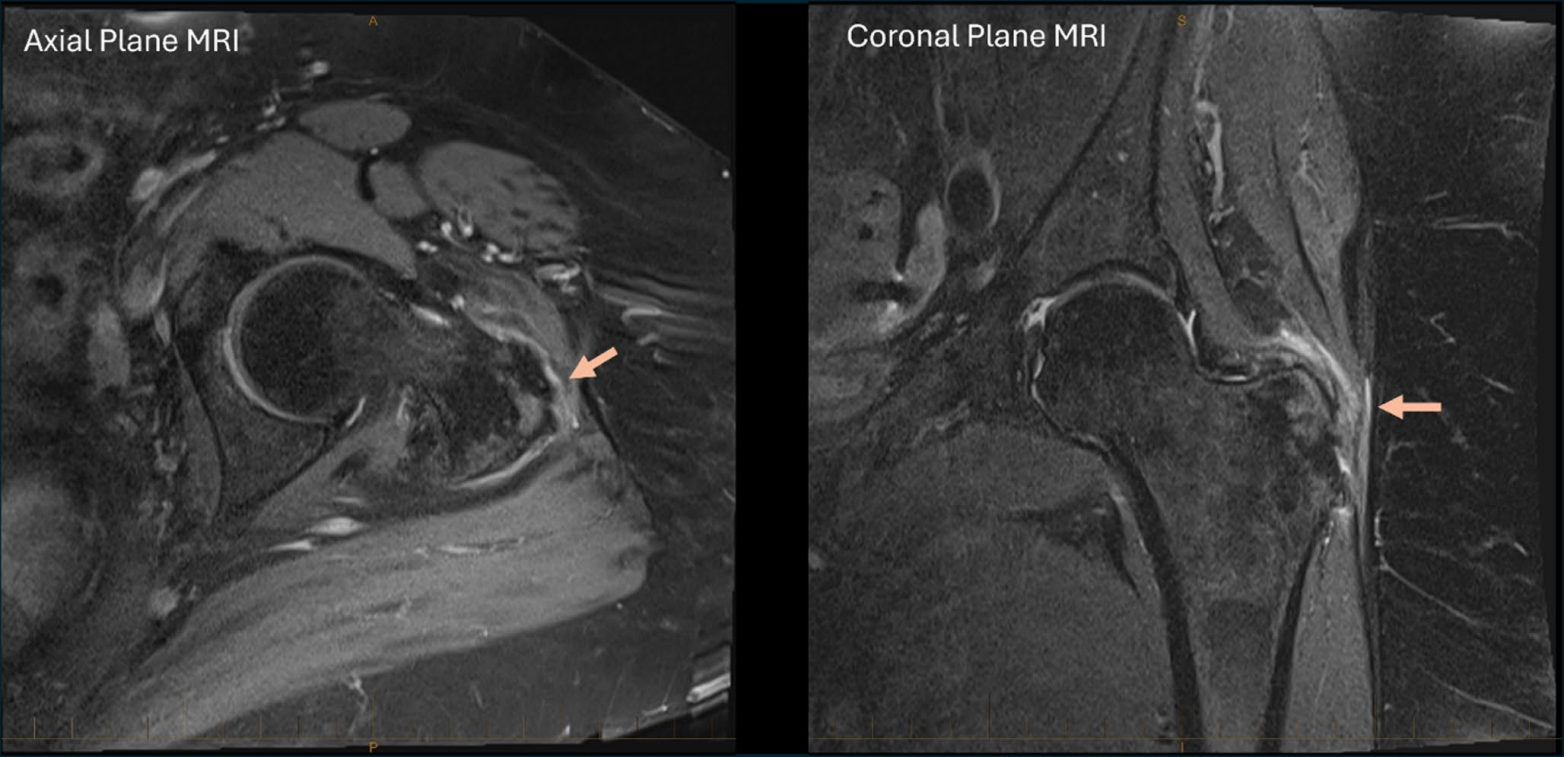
An example of tendon pathology in a participant of the LEAP trial demonstrated on axial and coronal plane T2-weighted MRI. Tears of the gluteus medius and minimus on the background of severe tendinopathy was reported
